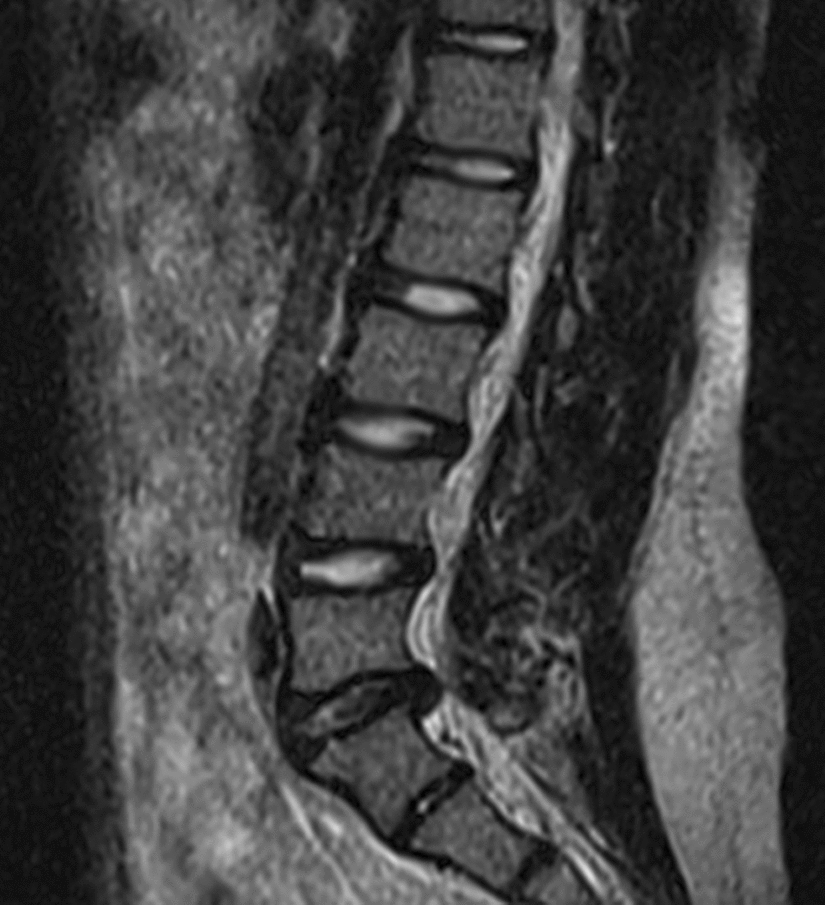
MRI of the lumbar spine obtained at the 13-year-old boy’s initial visit revealed platyspondyly of the lumbar vertebrae, and a lumbar disk herniation at the L5/S1 level
